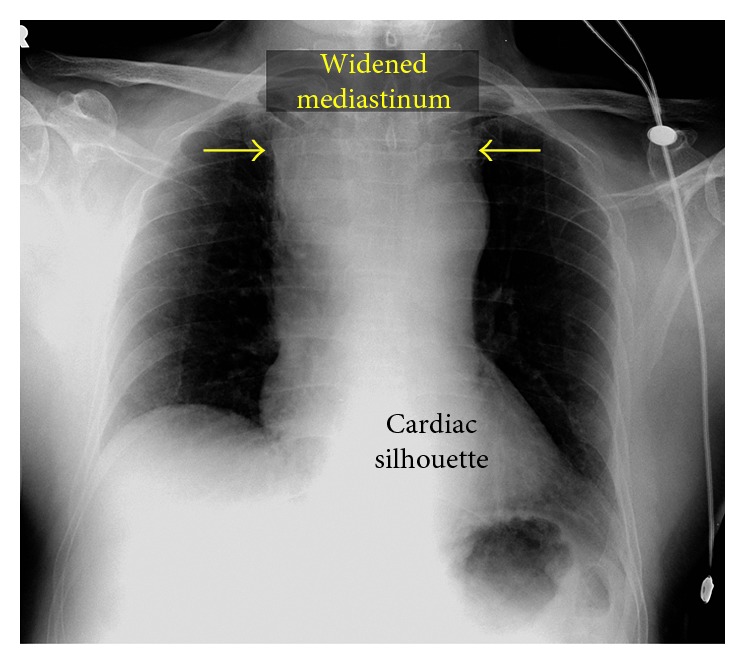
Chest radiography (AP orientation) demonstrating a widened superior mediastinum.
